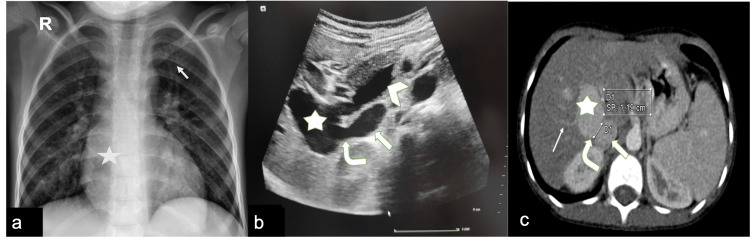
(A) Chest X-ray showing mild cardiomegaly (star) and prominent vascular marking (arrow). (B) Ultrasonography image showing side-to-side communication (curved arrow) between the main portal vein (star) and inferior vena cava (solid arrow) and also the patent superior mesenteric vein (arrowhead). (C) Corresponding CT scan image at a similar level showing side-to-side communication (curved solid arrow) between the main portal vein (star) and inferior vena cava (solid arrow), shunt size (line), and also the patent hypoplastic intrahepatic portal venous branches (simple arrow).
